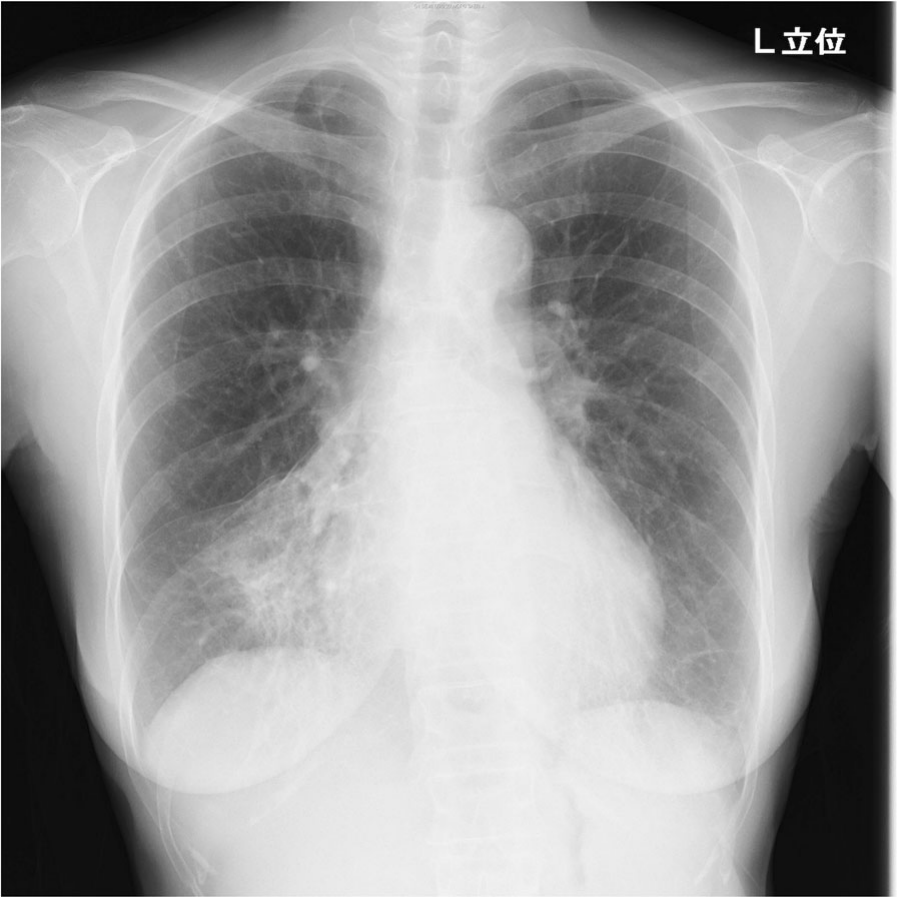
Chest x-ray image after the treatment
